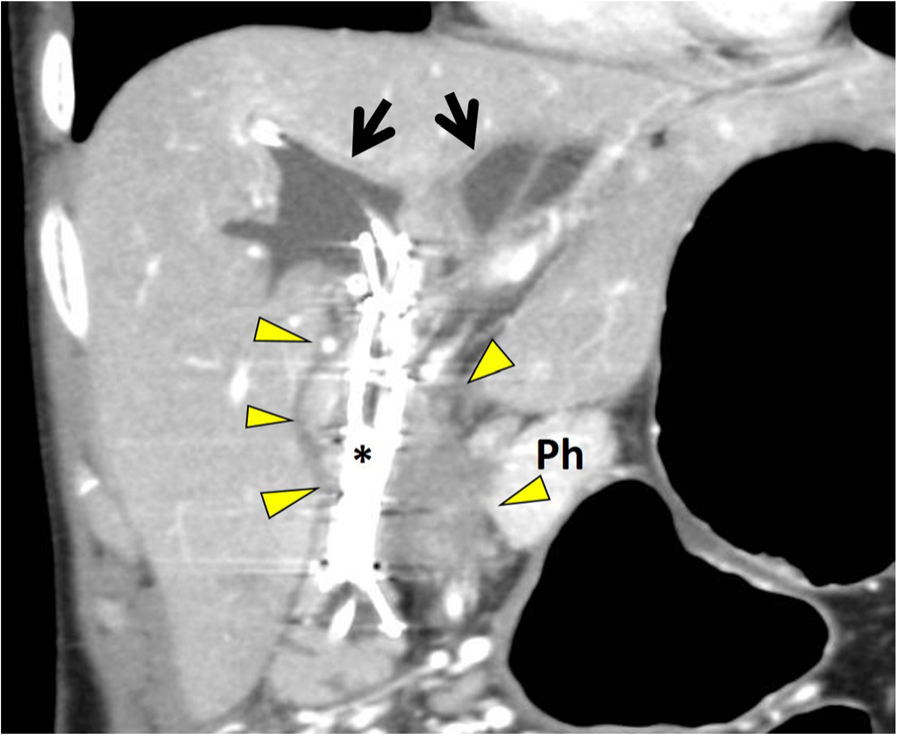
Computed tomography. A mass with an unclear and irregular border (*yellow arrow heads*) spreads from the hepatic hilum to the pancreatic head and invades the duodenum and the pancreatic head. The intrahepatic bile ducts were dilated (*black arrows*). *Ph* pancreatic head, *asterisk* metallic stent
